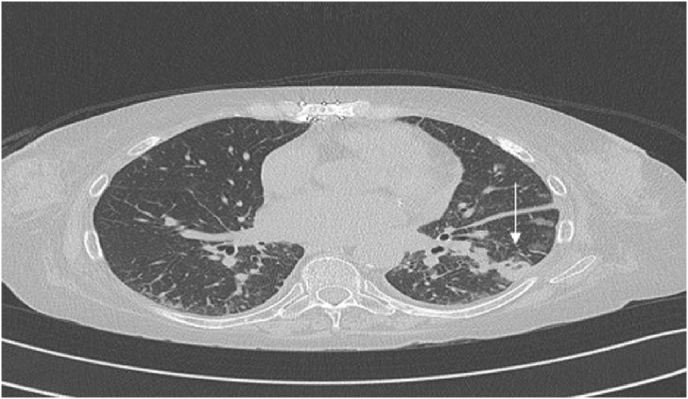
Chest CT showing the left lower lobe condensation (white arrow).
